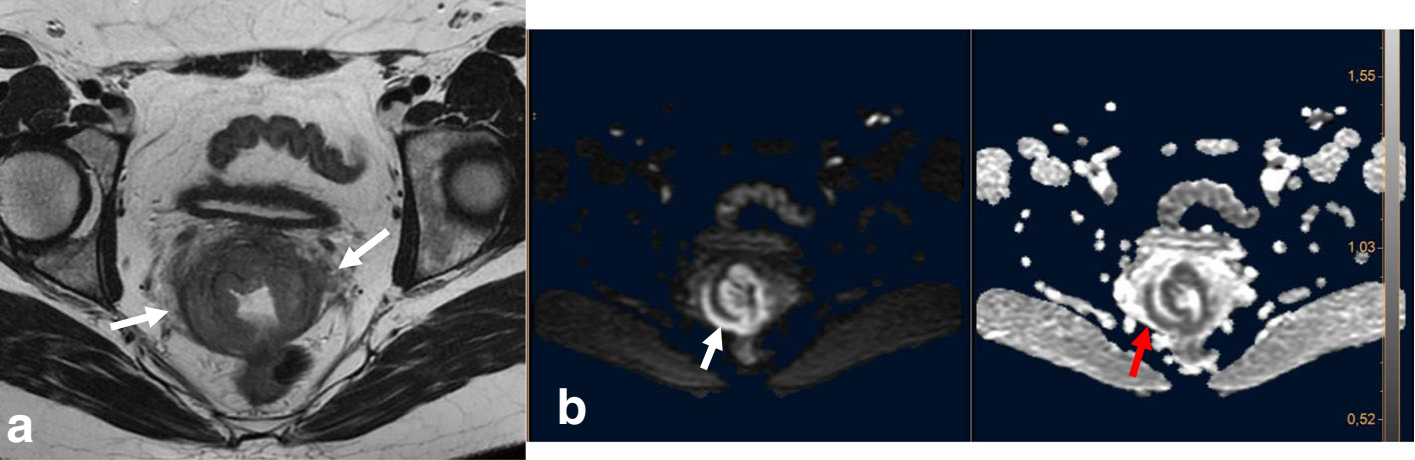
FIGO IB2 cervical cancer, with post-biopsy cervical edema. Biopsy was performed 2 days before MRI. a Axial oblique T2WI shows cervical cancer with apparent parametrial invasion (arrows). b DWI (*b* = 1000/ADC map) reveals a delineated tumor (arrows), without parametrial invasion
